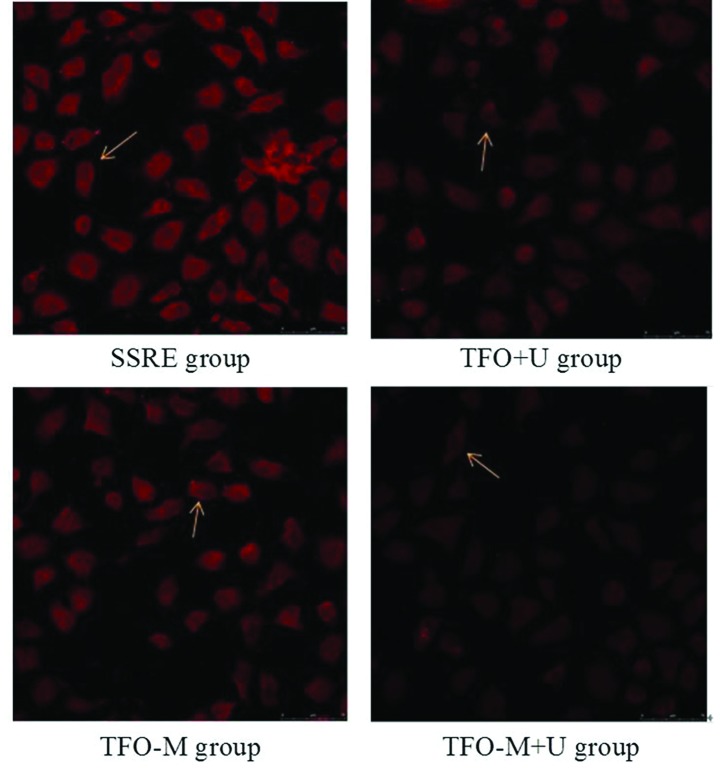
Immunofluorescent analysis of tissue factor protein *in vitro* (magnification, ×400). The arrows indicate positive immunofluorescent detection of tissue factor protein. TFO, triplex-forming oligonucleotides; M, microbubble; U, ultrasound; SSRE, shear stress response element.
